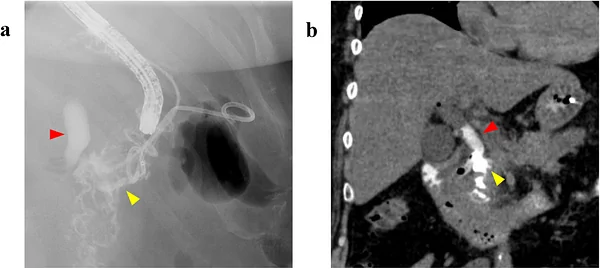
Fistula between the WON and the bile duct. Contrast medium was injected into the WON under endoscopic guidance. The red arrowhead indicates the bile duct, and the yellow arrowhead indicates the WON: (a) fluoroscopic image and (b) plain CT image. CT, computed tomography; WON, walled‐off necrosis.
